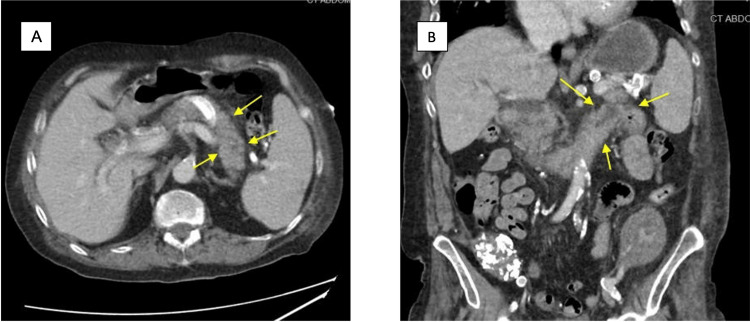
CT scan images of transverse (A) and coronal (B) sections showing edematous body and tail of pancreas (yellow arrows).
